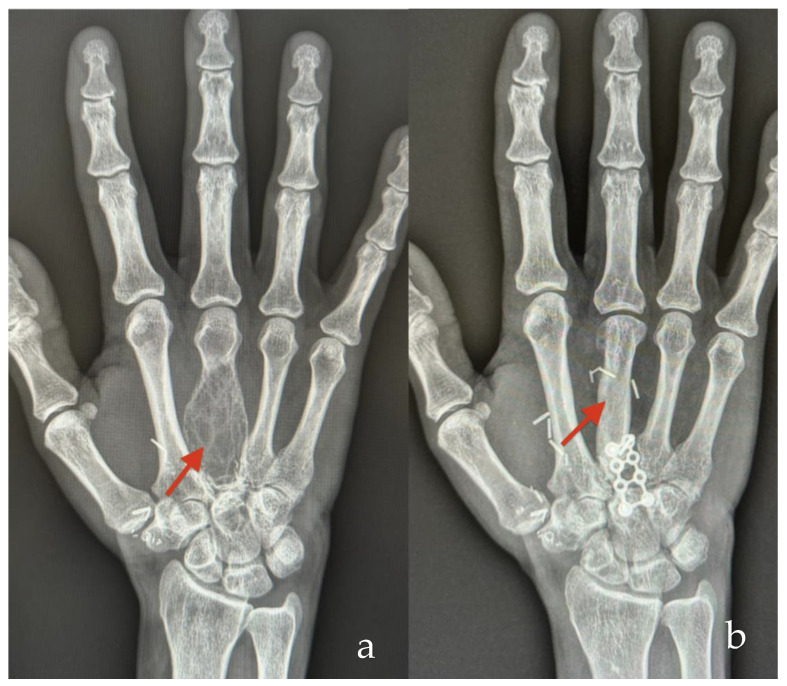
Female patient who underwent vascularized bone reconstruction due to resection of giant cell tumor (red arrow) of her right hand. (a) Preoperative X-ray—recurrence of giant cell tumor of the metacarpal bone. (b) Postoperative X-ray—one year after metacarpal bone reconstruction with free MFC bone flap (red arrow) assisted with 3D technology.
